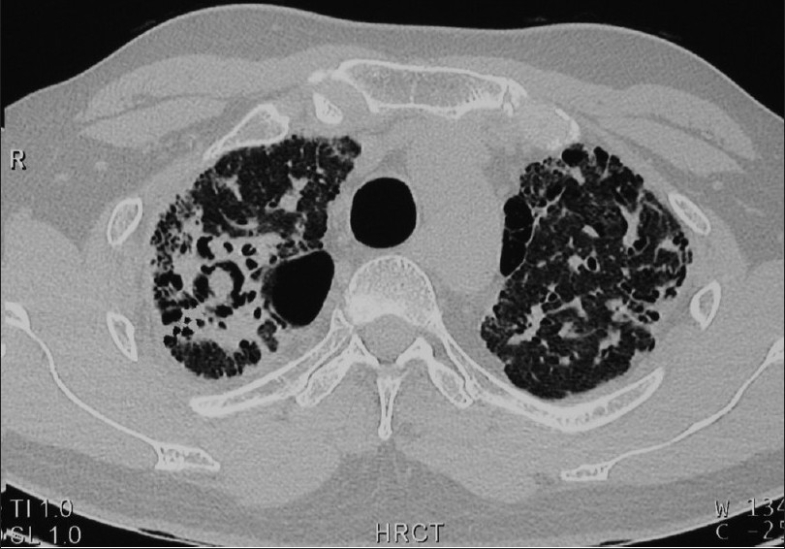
HRCT slide (2007) showing aspergilloma in the right upper lobar cavity and extensive fibrosis in both lung fields
